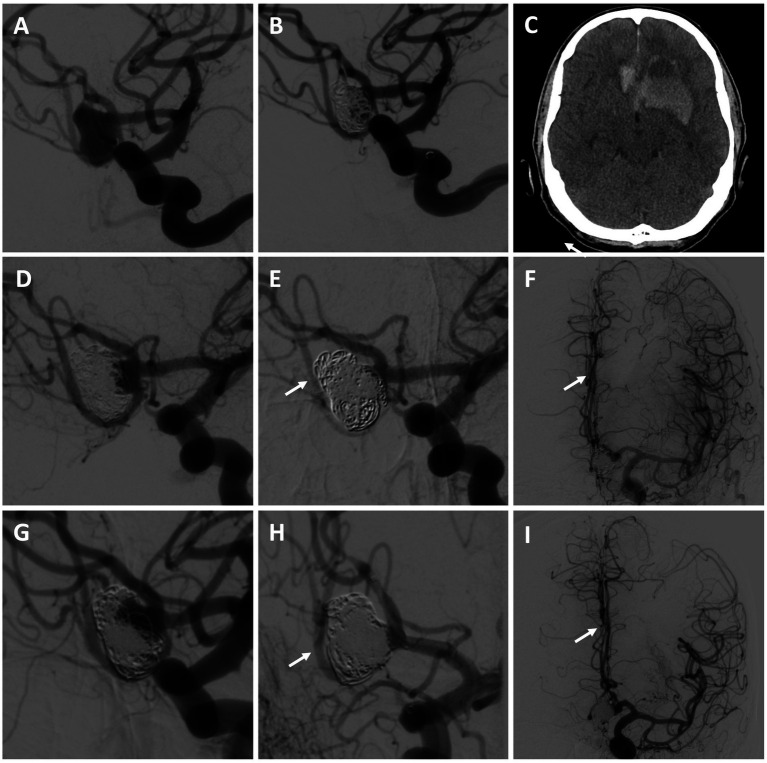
Patient #8. A 61-year-old male presented with SAH and intraparenchymal hemorrhage from a previously embolized Acomm aneurysm arising from an unpaired left A1 [(A) left ICA angiogram demonstrating initial Acomm aneurysm, and after coil embolization (B); (C) head CT demonstrating SAH and IPH]. A repeat coil embolization was performed, leaving a neck residual to preserve the Acomm [(D) post re-coiling angiogram demonstrating a neck residual and patent Acomm]. An A3-A3 bypass was performed, followed by repeat aneurysm embolization with Acomm sacrifice [(E,F) post-treatment angiogram demonstrating no residual aneurysm and filling of the right ACA through the bypass (highlighted by arrows)]. (G) 3-month repeat angiogram demonstrated aneurysm re-growth and an open Acomm, treated with flow diversion across the left A1-2 and repeat aneurysm coiling (including Acomm re-sacrifice) [(H,I) post-treatment angiogram demonstrating no residual aneurysm and filling of the right ACA through the bypass (highlighted by arrows)]. On follow up, the bypass was open and there was no aneurysm recurrence. The patient was neurologically intact and living independently.
